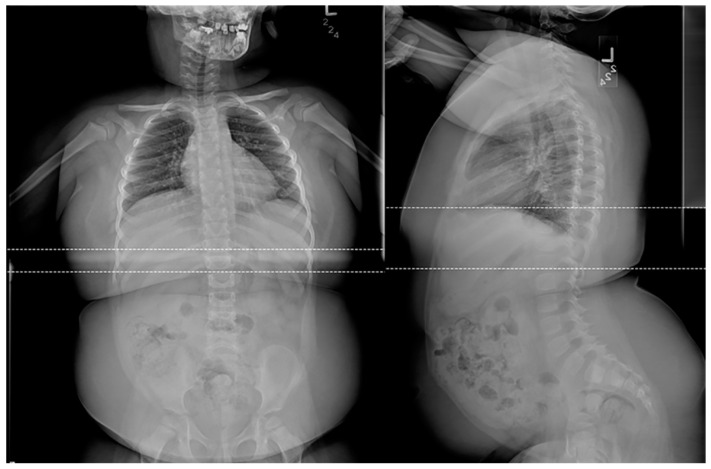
Frontal and lateral radiographs of a 4-year-old female patient with confirmed Prader-Willi syndrome show a significant adipositas, gracile, overtubulated humeri, and significant dental work secondary to advanced dental decay.
